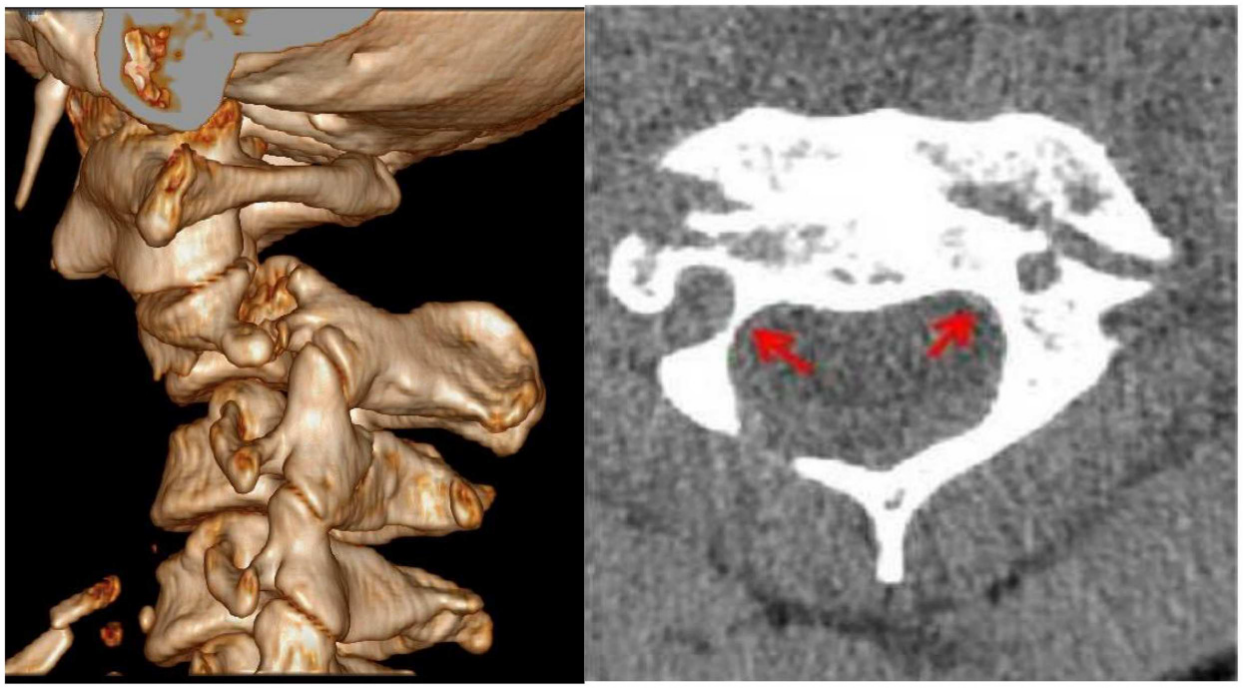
Preoperative cervical spine CT three-dimensional reconstruction and plain scan. The three-dimensional reconstruction showed Hangman fracture, and the axial view showed the high-riding vertebral artery, as a height of the isthmus internal marked by the left red arrow.
